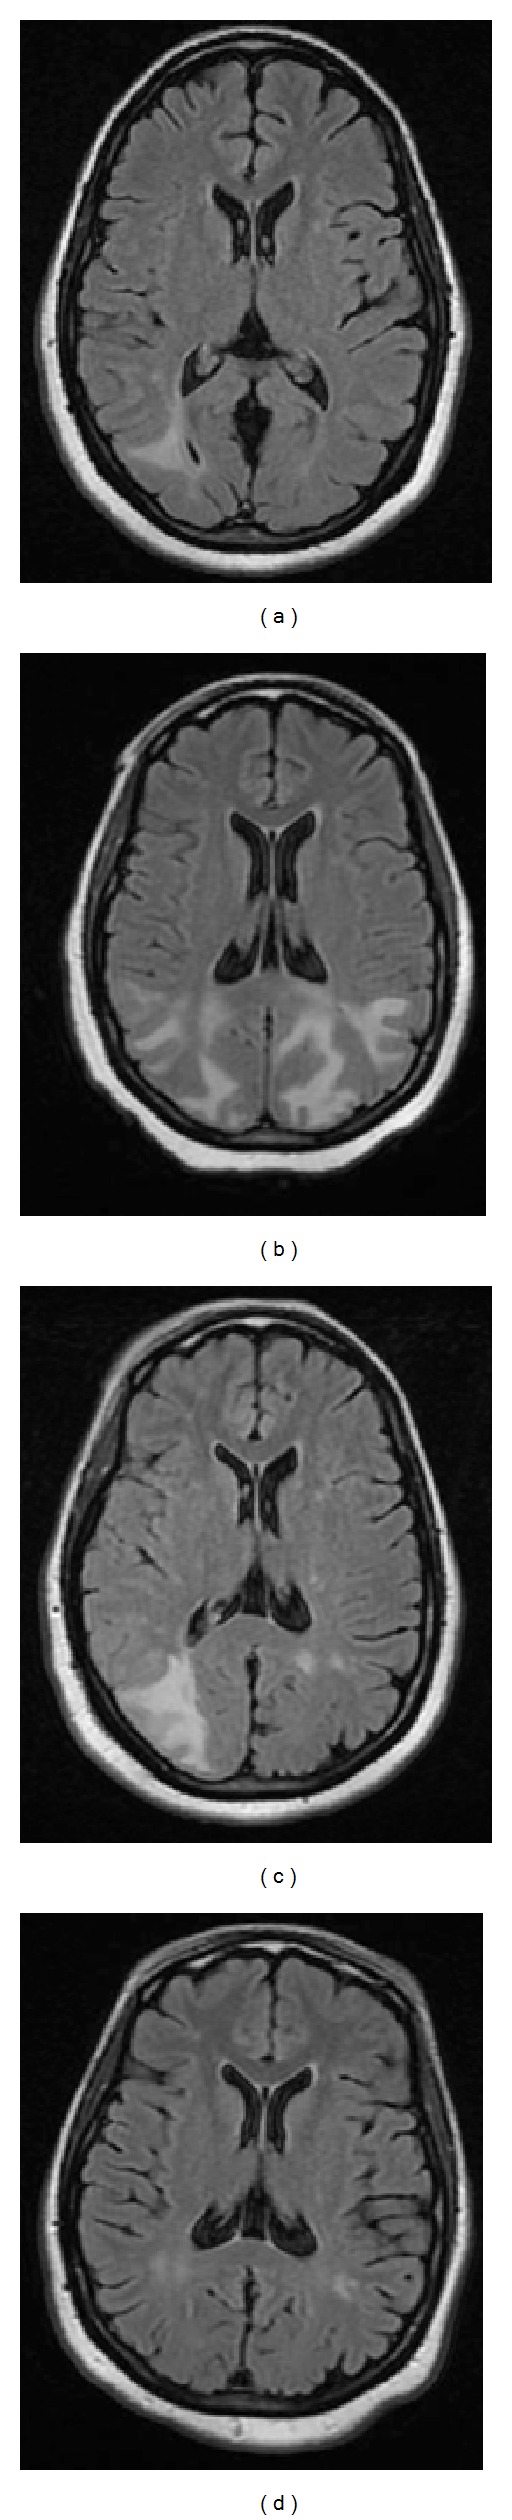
(a) At presentation: axial T2-weighted fluid-attenuated inversion recovery MRI showed multiple large areas of edema in the occipitoparietal regions. (b) At presentation: axial T2-weighted FLAIR MRI showed multiple large areas of edema in the occipitoparietal lobe, with the right greater than the left with no mass effect. (c) At 2-week followup: axial T2-weighted FLAIR MRI showed resolving edema. (d) At 5-month followup: axial T2-weighted FLAIR MRI showed no further edema.
